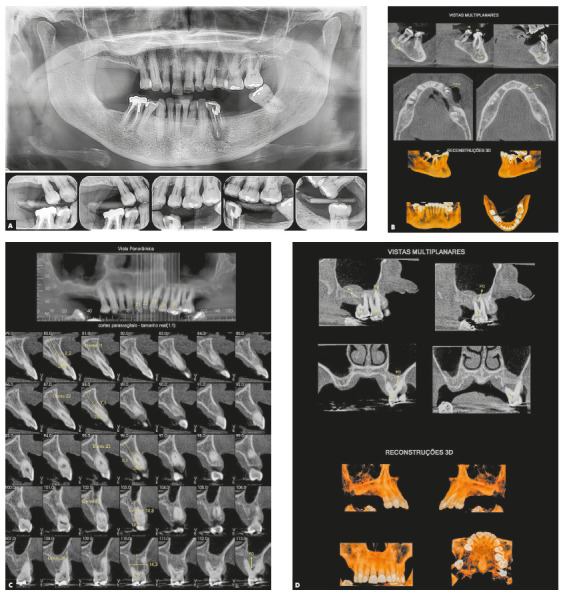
A) Initial radiographs of Case 2. B-D) Initial tomographic sections of areas of interest of Case 2.
